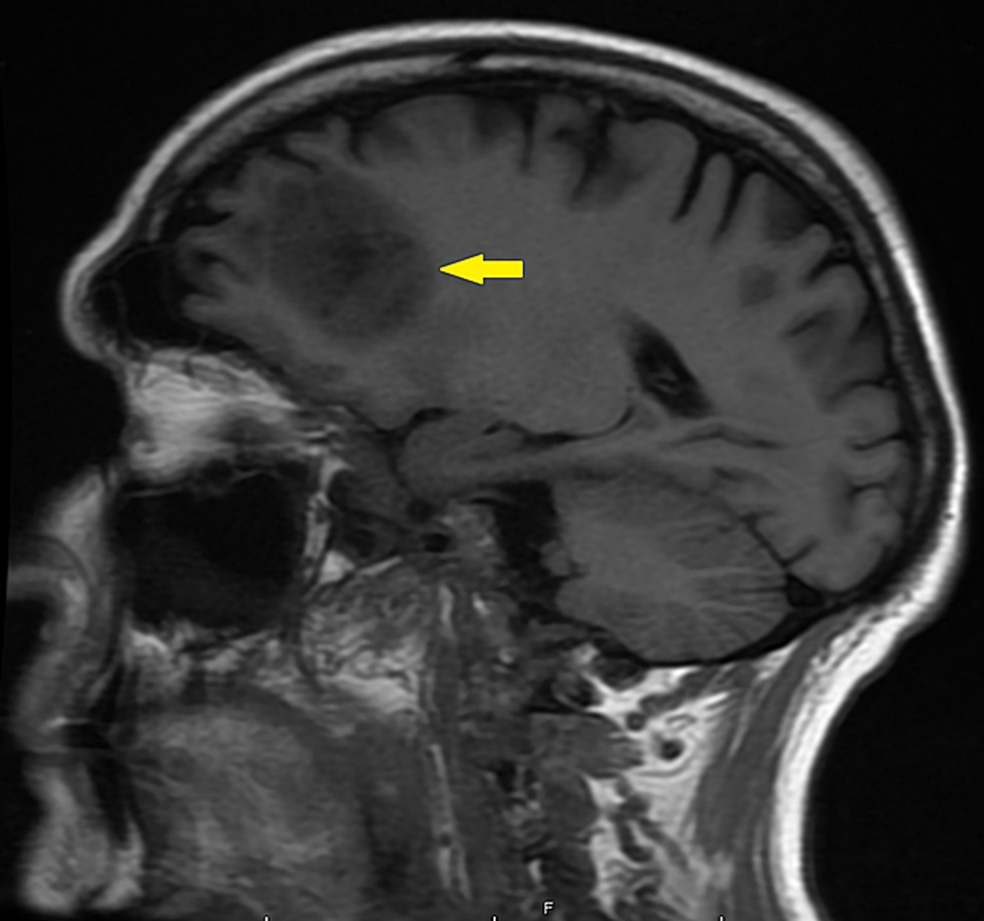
MRI brain with contrast showing mass-like peripherally enhancing lesions in the left frontal lobe.
